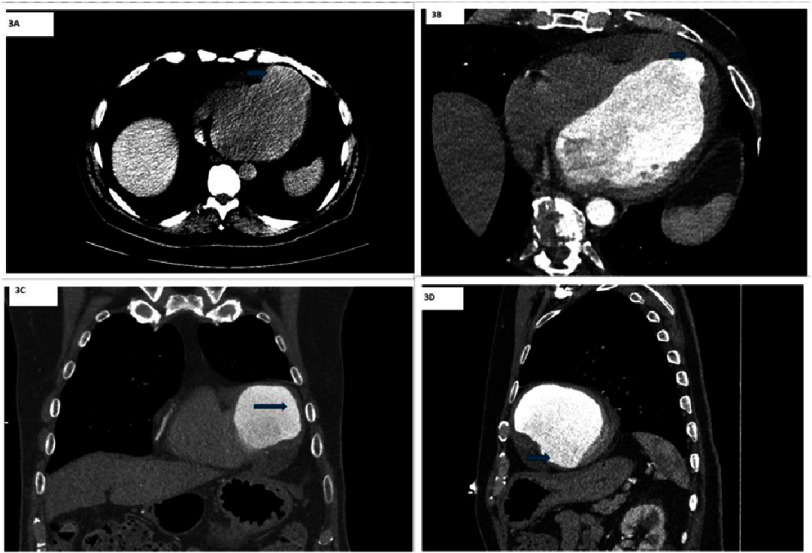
Contrast-enhanced CT of the chest and aorta reveals focal dilatation at the left ventricular apex (3A, 3B, 3C, and 3D) with a narrow base and abrupt transition, raising suspicion for a left ventricular pseudoaneurysm.
